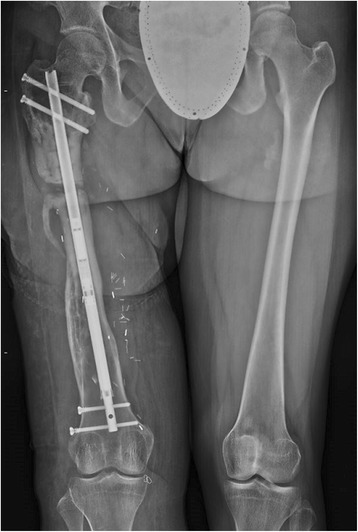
Long leg weight-bearing plain films demonstrating femur at eight weeks after lengthening with mechanical shortening of nail
